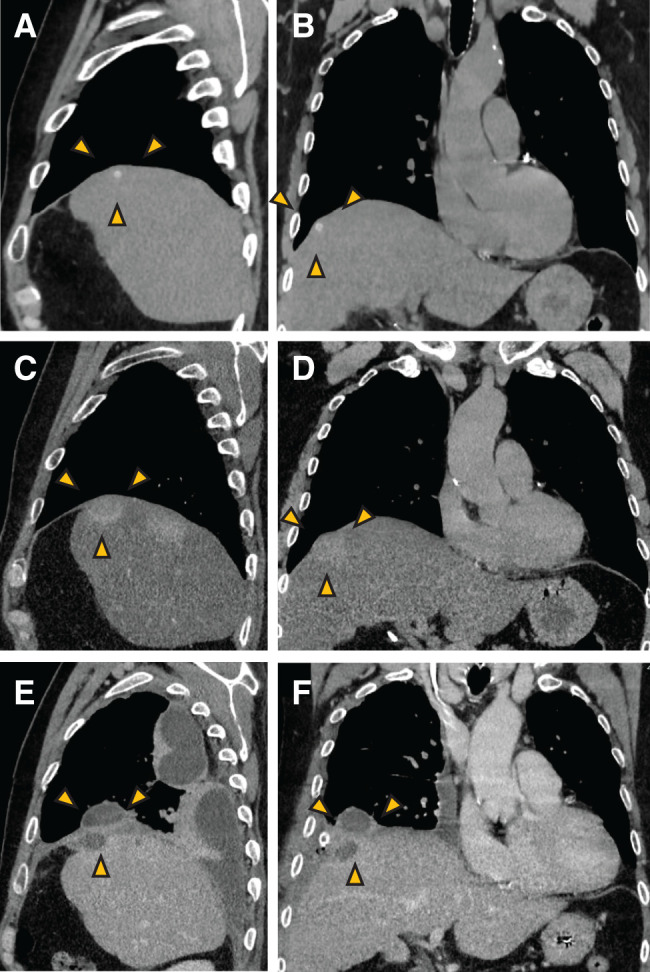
Course of preoperative CT images. (A, B) CT images 1 month after cholecystectomy. The images showed residual gallstone on the liver surface. The residual gallstone is indicated by yellow arrowheads. (C, D) CT images 6 months after cholecystectomy showing a HDA in the liver. The HDA is indicated by yellow arrowheads. (E, F) CT images 1 year after cholecystectomy. The images showed a right subdiaphragmatic fluid space suspicious of an intra-abdominal abscess and a pleural effusion suspicious of a right pyothorax. These areas are indicated by yellow arrowheads. HDA, high-density area
